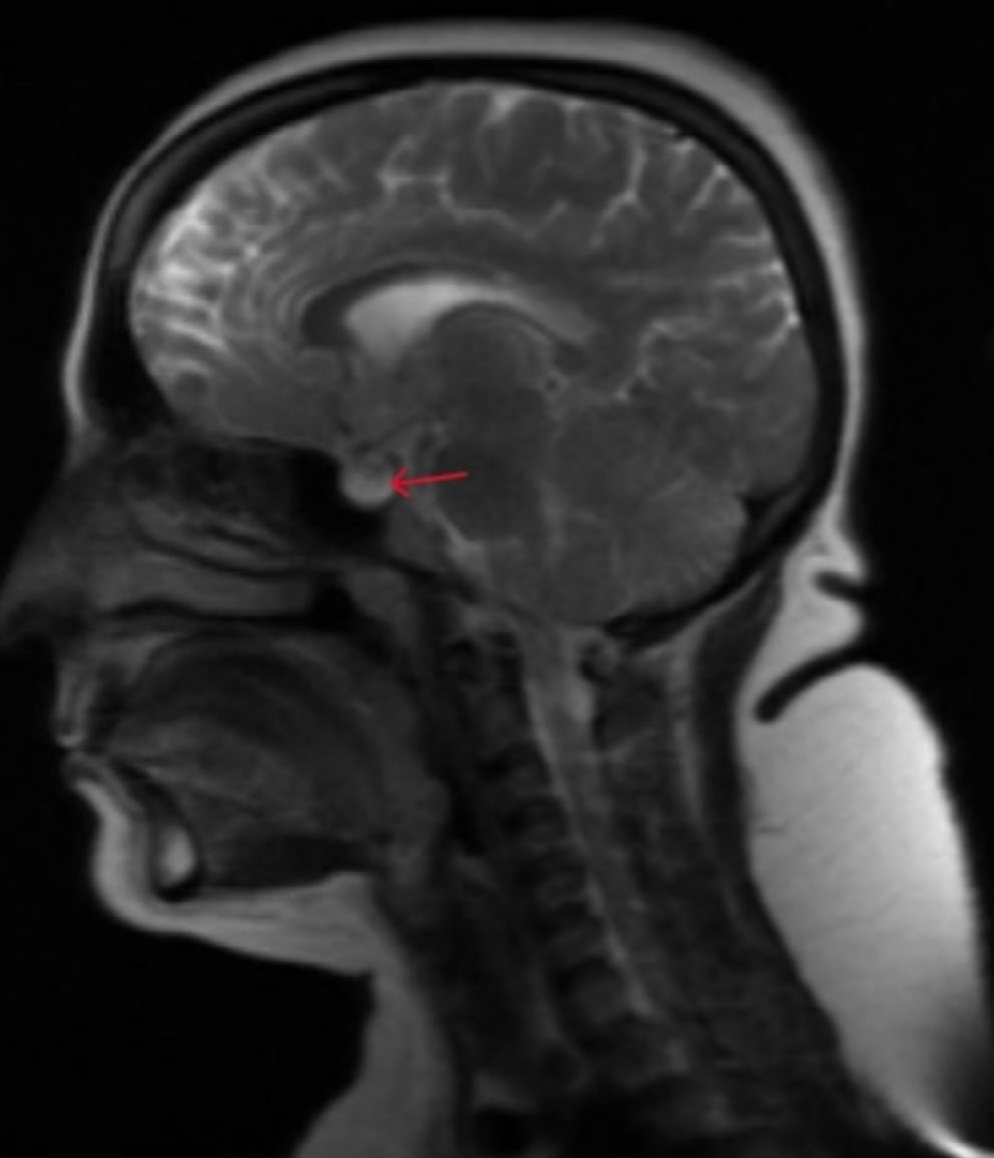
MRI brain sagittal view T2 sequence shows empty sella as indicated by the red arrow.
